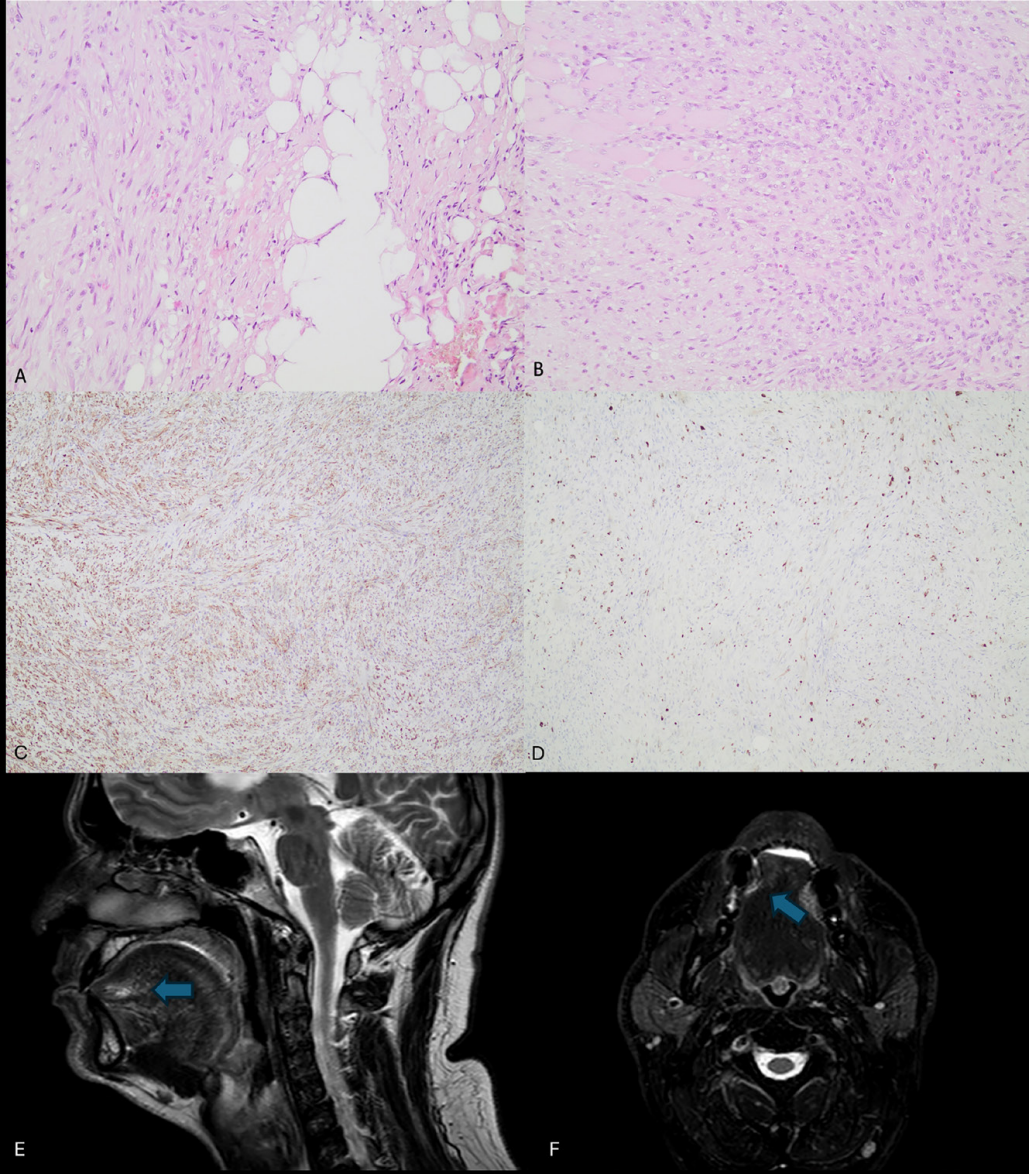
(A, B) Spindle cell proliferation with low-to-moderate nuclear atypia, infiltrating adipose tissue (A, HE X200), and muscle fibers (B, HE X200). (C) The neoplastic cells show diffuse positivity for SMA (X100). (D) The Ki-67 proliferative index is approximately 15-20% with heterogeneous expression (X100). (E, F) Magnetic Resonance Imaging of the head and neck region. The lesion is featured with an increase in T2 signal.
